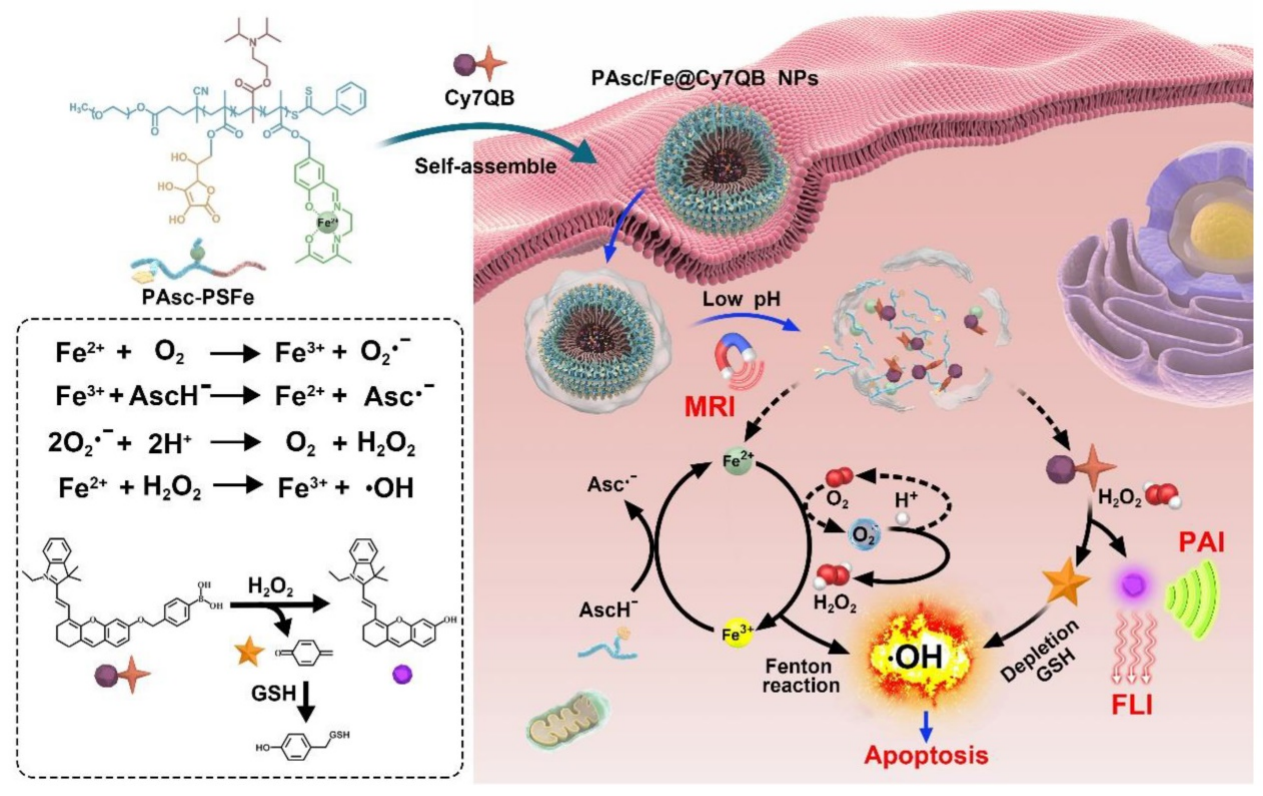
Fabrication procedure and scheme of the theranostic mechanism of PAsc/Fe@Cy7QB for MRI/FLI/PAI multi-modal imaging and the H_2_O_2_-catalyzed •OH burst cascade.
